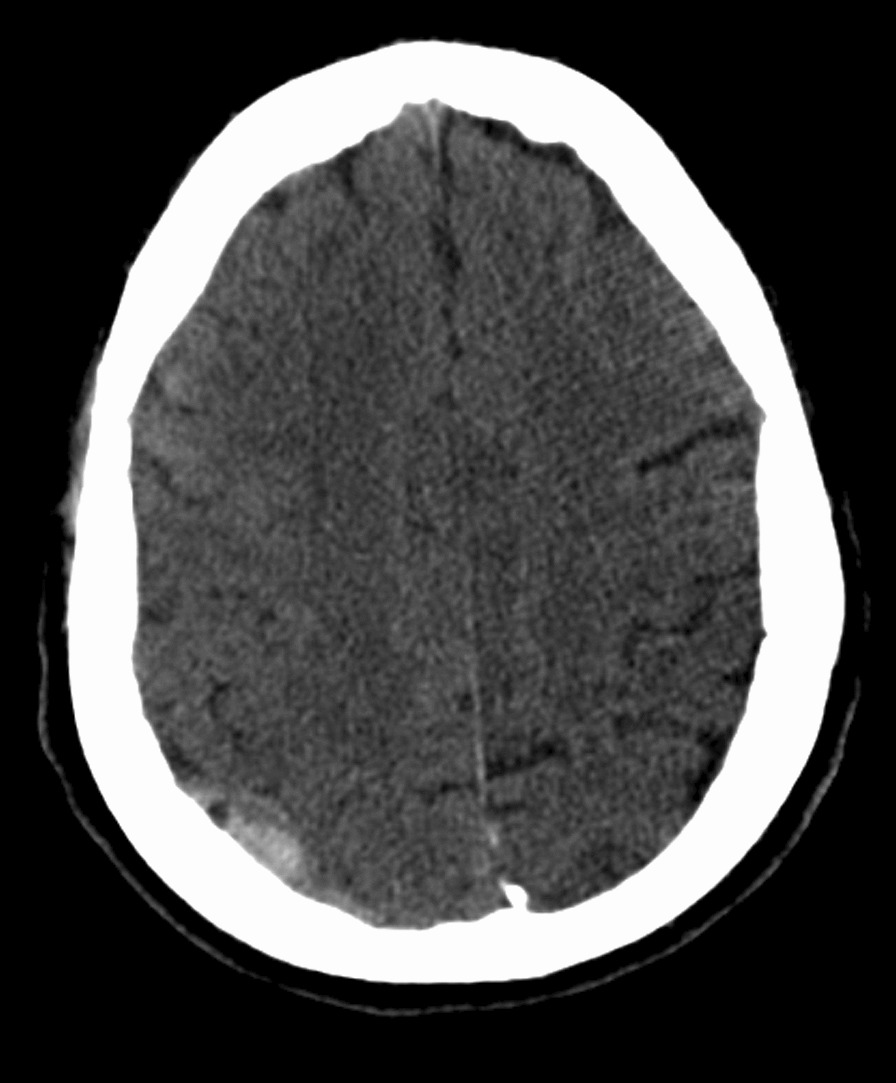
Computed tomography of the head demonstrating interval stable epidural hematoma
